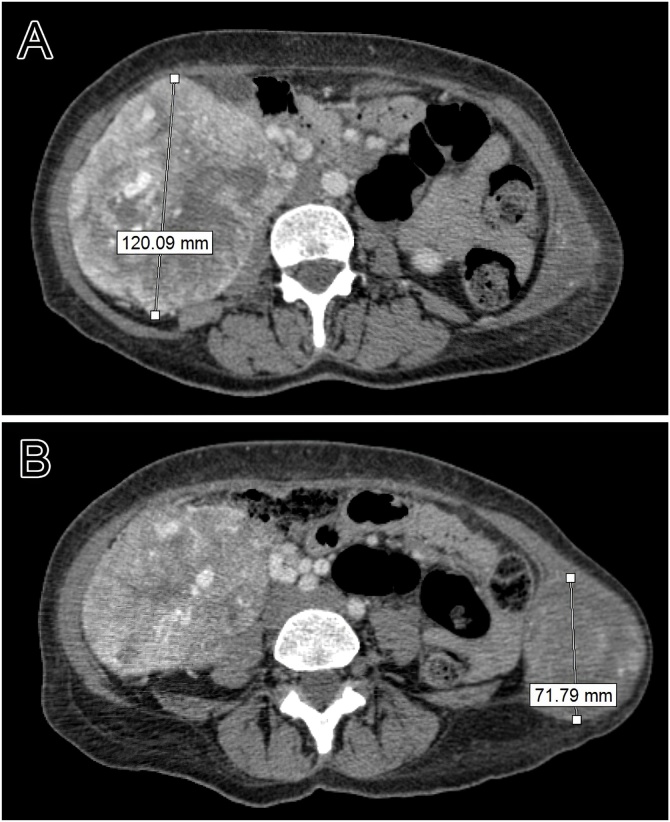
Contrast abdominal CT revealing mass in the right kidney (A) and a mass in the left abdominal wall (B).
